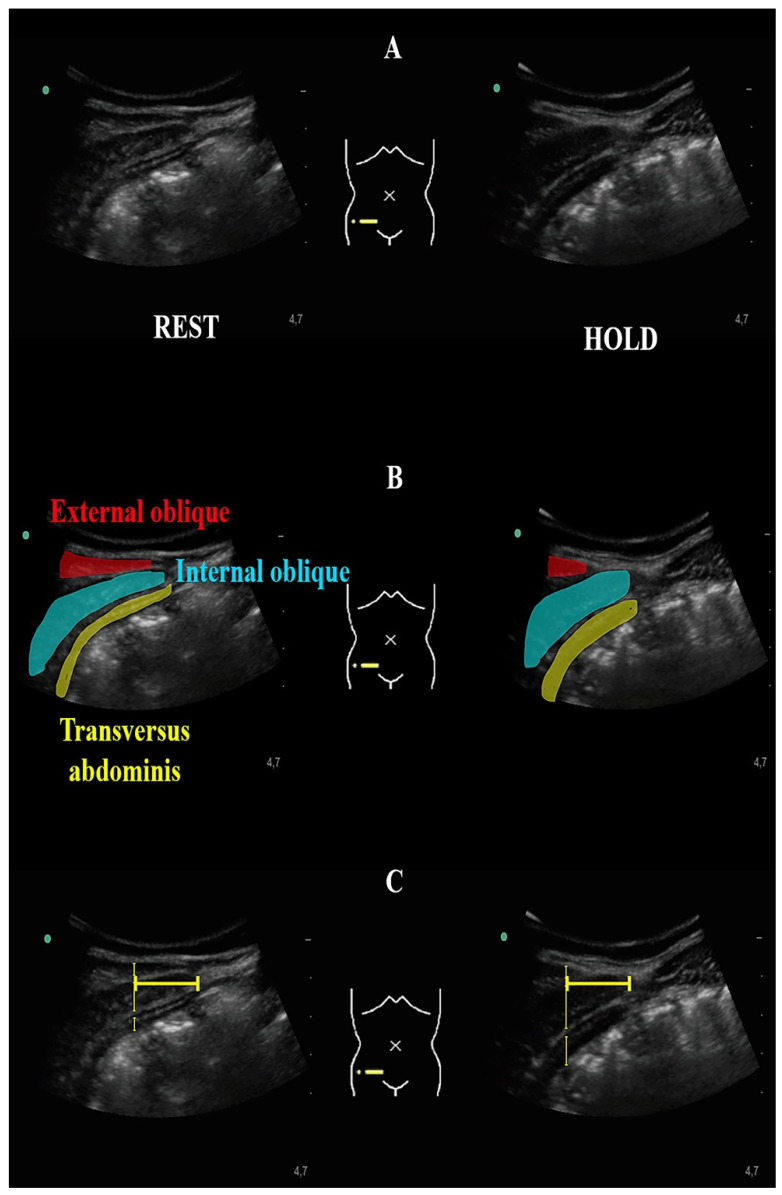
Ultrasound image of the deep abdominal muscles (A); Color mark of the deep abdominal muscles (B); Ultrasound measurements conducted in a vertical line located 3 cm lateral to the rectus sheath muscle-fascia junction (semilunaris line) (C).
